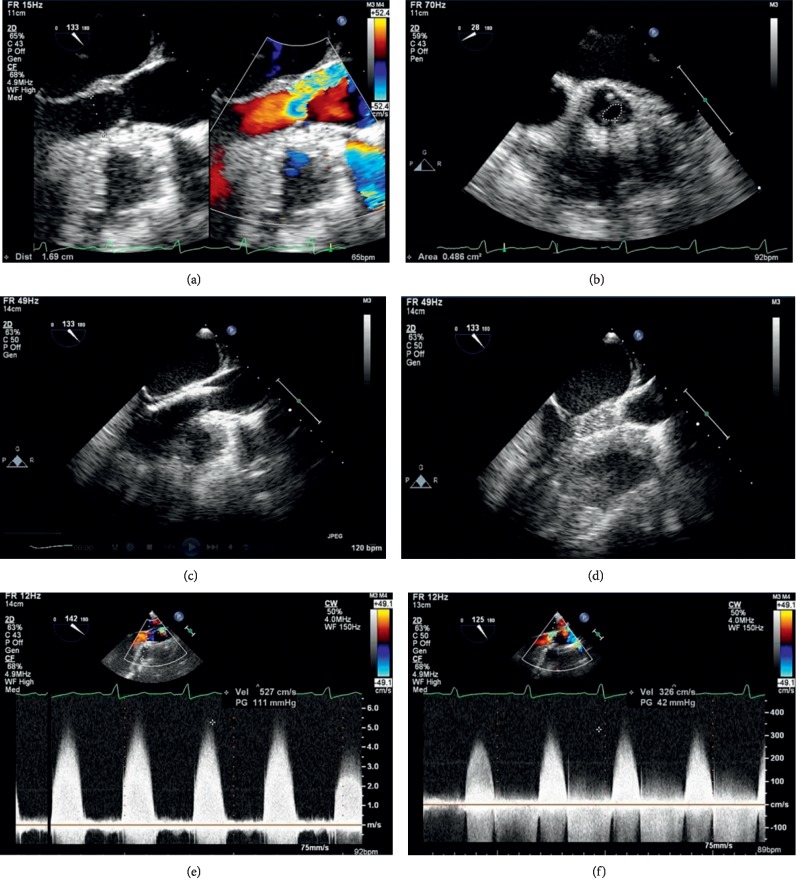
Representative images obtained from a patient during transthoracic ultrasound-guided percutaneous balloon aortic valvuloplasty. (a) Preoperative measurement of the aortic annulus (1.69 cm). (b) Preoperative measurement of the aortic valve area (0.48 cm^2^). (c) Intraoperative echocardiography showed that the guide-wire had passed through the stenotic aortic valve. (d) Intraoperative echocardiography showed that the balloon had passed through the stenotic aortic valve and the balloon had filled to expand the aortic valve. (e) The preoperative aortic valve flow rate was 5.3 m/s, and severe stenosis was evident. (f) Postoperatively, the aortic valve flow rate had decreased to 3.2 m/s and the aortic stenosis was significantly relieved.
